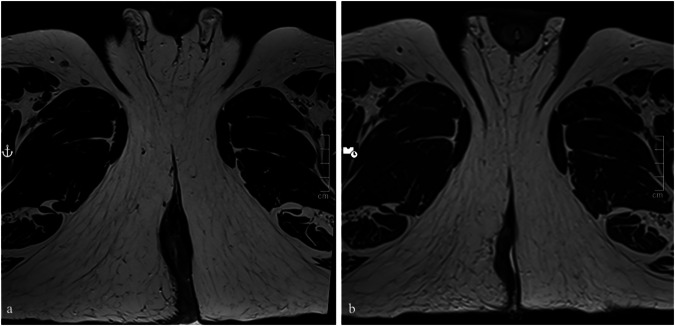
Turbo spin-echo T2-weighted paraxial images demonstrate residual disease after combination CRT before (a) and after treatment (b): the patient was classified as a non-CR case
